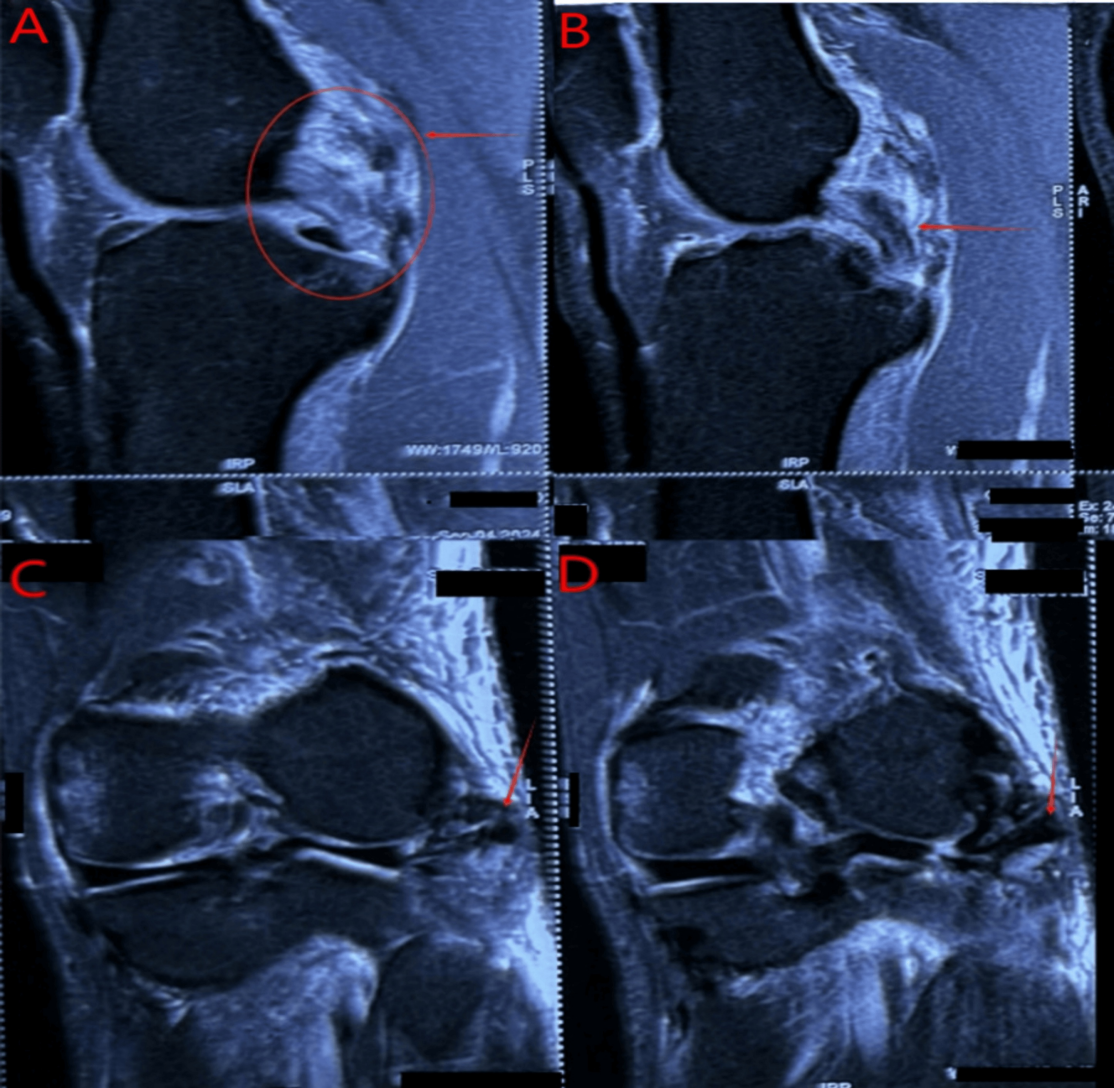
MRI of left knee showing ACL tear and PLC avulsion injury (two sagittal and two coronal views) (A) Sagittal view showing the area at the intercondylar notch (red circle with arrow) with the absence of ACL fibers. (B) Sagittal view of the intercondylar notch showing the buckling of PCL (indirect sign of ACL tear). (C) Coronal view showing PLC avulsion injury (the lack of continuous fibers of the lateral collateral ligament). (D) Coronal view showing PLC avulsion injury with avulsed lateral collateral ligament MRI, magnetic resonance imaging; PLC, posterolateral corner; PCL, posterior cruciate ligament; ACL, anterior cruciate ligament
